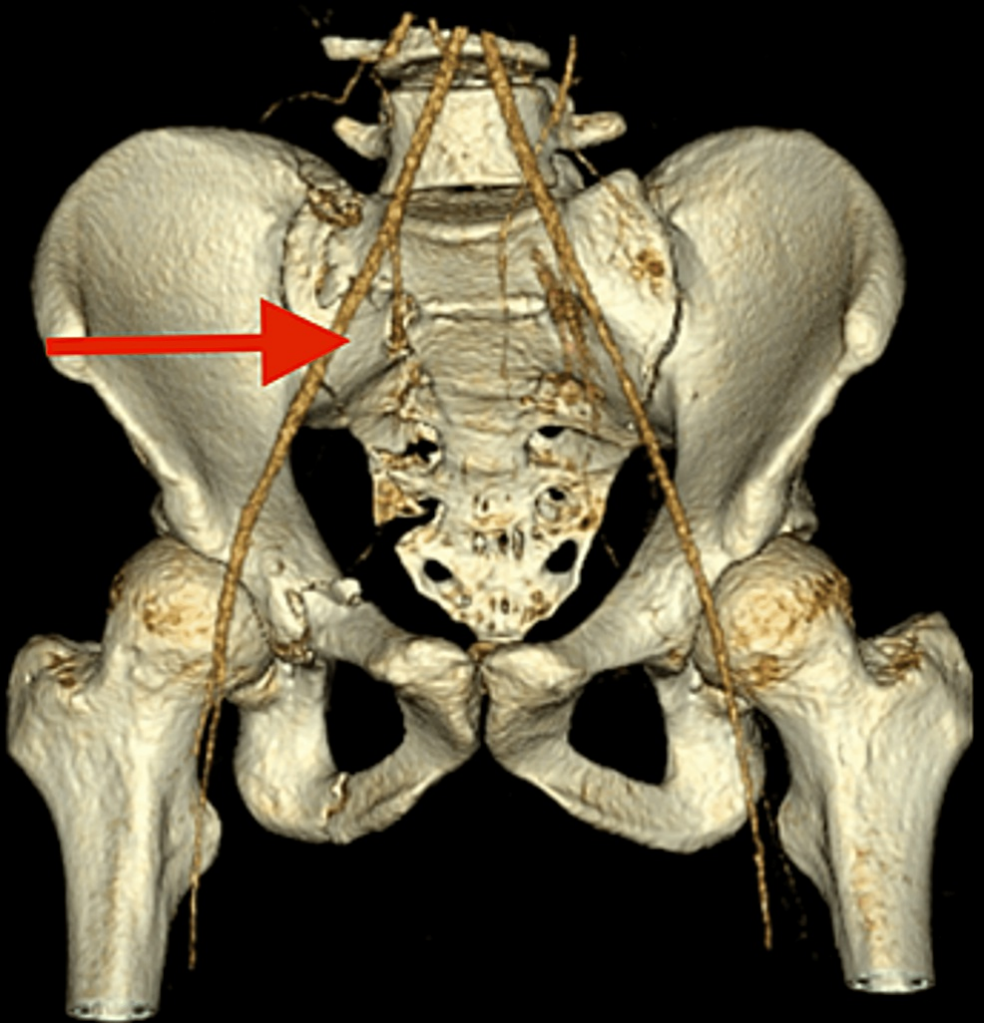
CT scan of the second patient showing right sacral ala fracture with ipisilateral superior and inferior pubic rami fracture.
